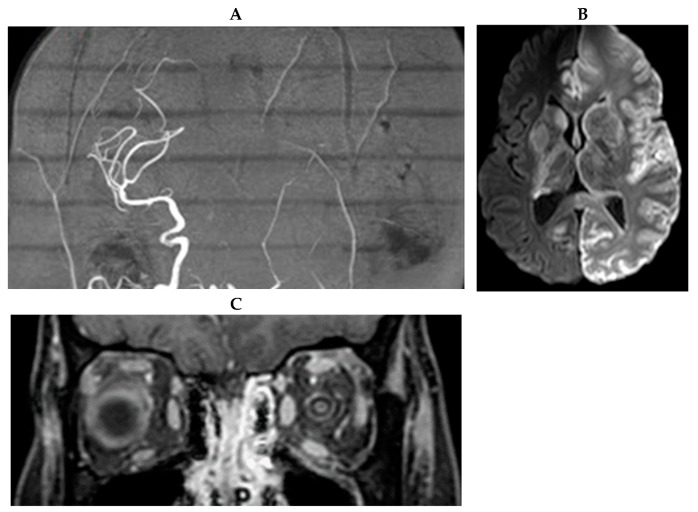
Angiography and magnetic resonance (MR) imaging on day +2. (A), TOF-angiography reveals perfusion only via the right internal carotid artery and middle cerebral and proximal parts of the basilar artery (BA). (B), DWI/ADC-Map showing extensive diffusion restriction of the entire left hemisphere and the right basal ganglia. (C), coronar T1w-mDIXON MRI post administration of gadolinum documenting the persistent swelling of the left optic nerve and the left intraorbital eye muscles.
